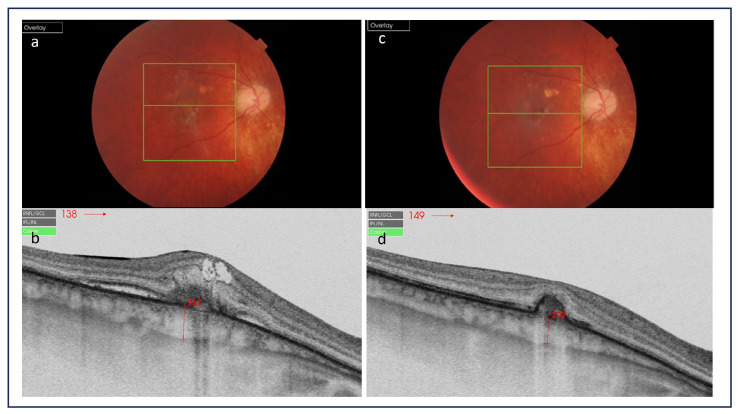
(a,c) Color pictures of the right eye diagnosed for punctate inner choroidopathy; (b,d) optical coherence tomography scans demonstrate choroidal thickness (CT) changes under inflammatory choroidal neovascularization before and after the treatment—the “sponge sign”. Before treatment, CT corresponded to 257 µm and then decreased to 202 µm after intravitreal bevacizumab injection.
